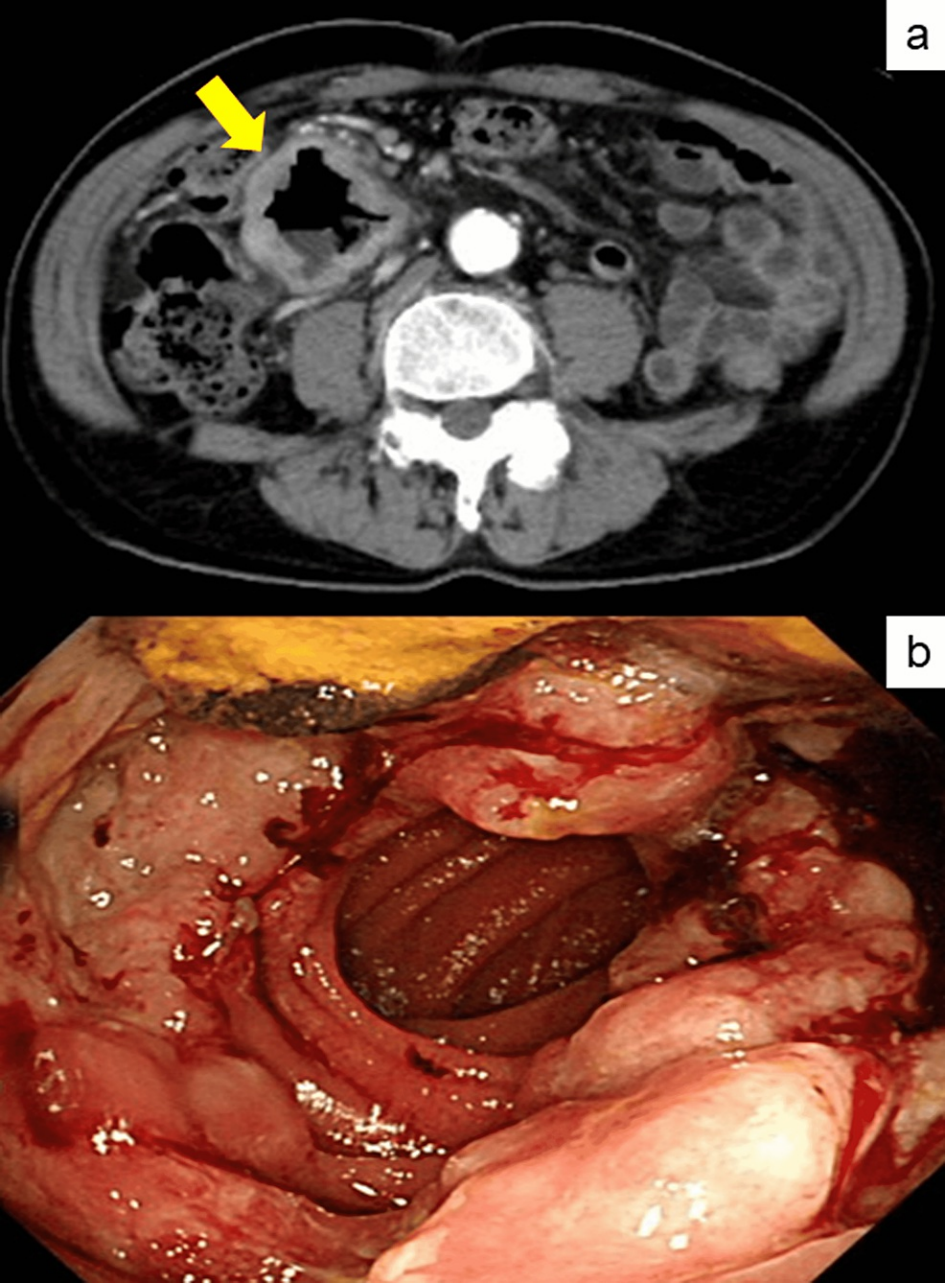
Clinical imaging. (a) CT scan showed a 6.5-cm aneurismally dilated mass in the small bowel (yellow arrow). (b) Capsule endoscopy revealed a large circumferential hemorrhagic mass in the jejunum.
